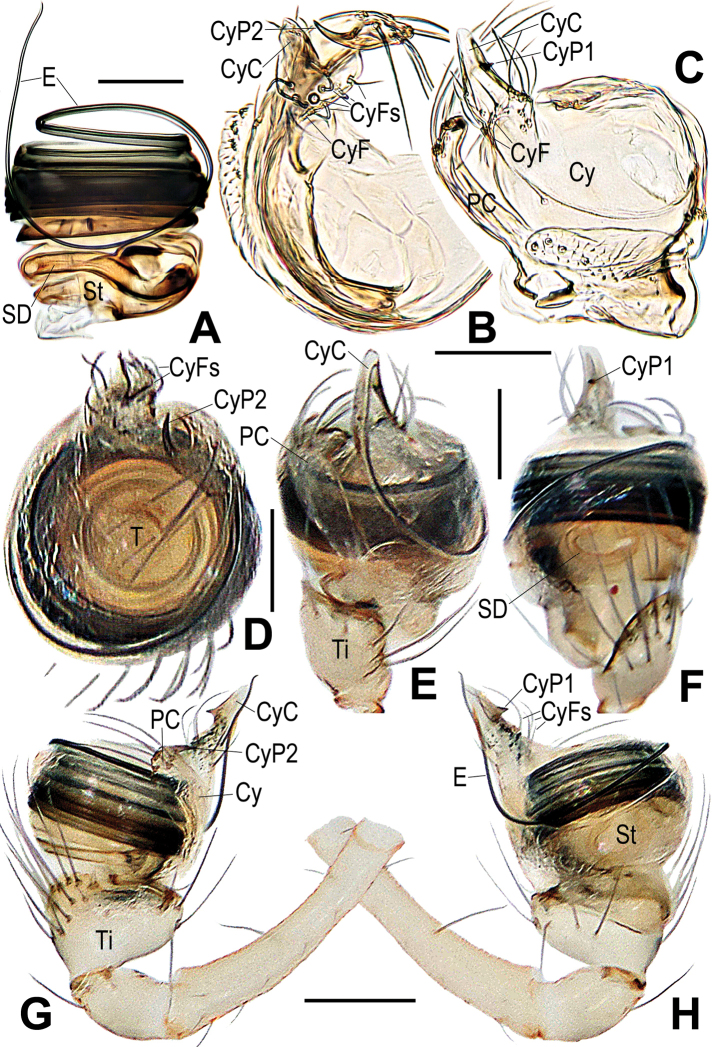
Chimenataiwanica* (Ono, 2007) comb. nov., male A left palpal bulb, retrolateral B cymbium, apical C cymbium, dorsal-retrolateral D left palp, apical E left palp, ventral F left palp, dorsal G left palp, retrolateral H left palp, retrolateral. Abbreviations: Cy cymbium; CyC cymbial conductor; CyF cymbial fold; CyFs setae on cymbial fold; CT cymbial tooth; CyP2 process on paracymbium; PC paracymbium; E embolus; SD spermatic duct; St subtegulum; T tegulum; Ti palpal tibia. Scale bars: 0.10 mm.
